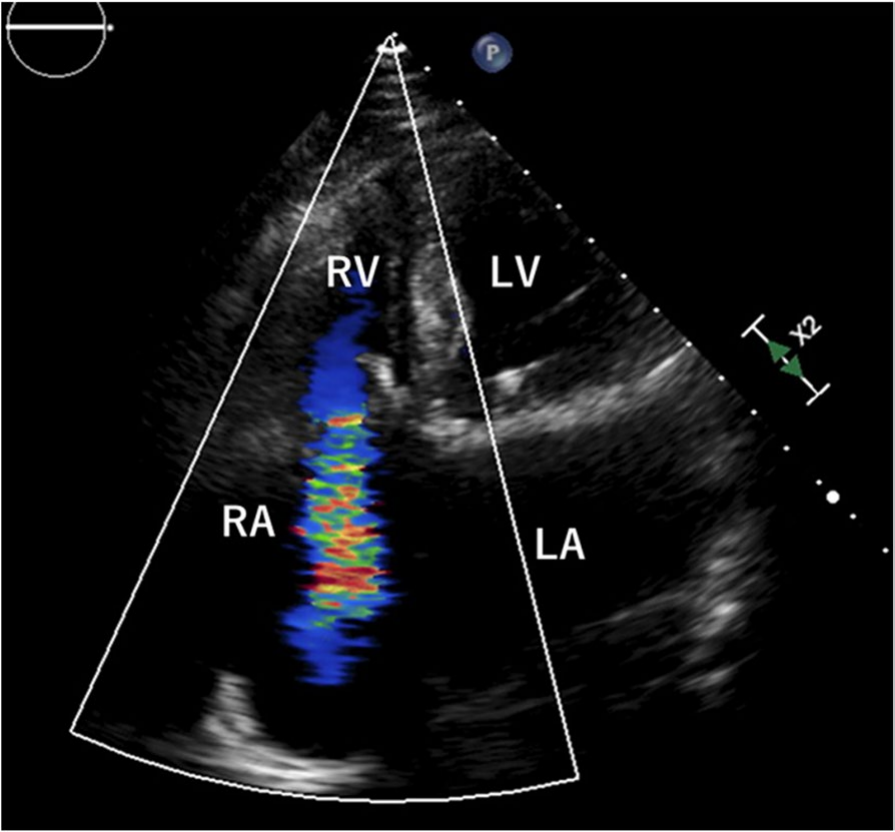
Preoperative transthoracic echocardiography shows severe transvalvular leakage after tricuspid valve replacement. *LA* left atrium, *LV* left ventricle, *RA* right atrium, *RV* right ventricle
